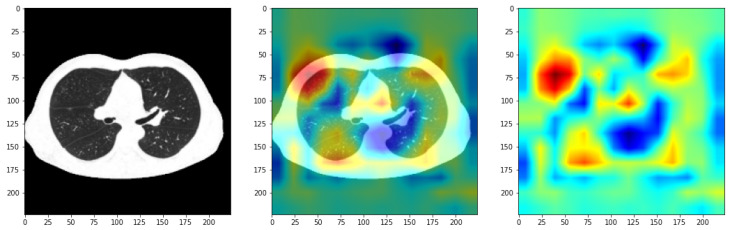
CAM applied to preprocessed COVID-19 CT using VGG16 model.
